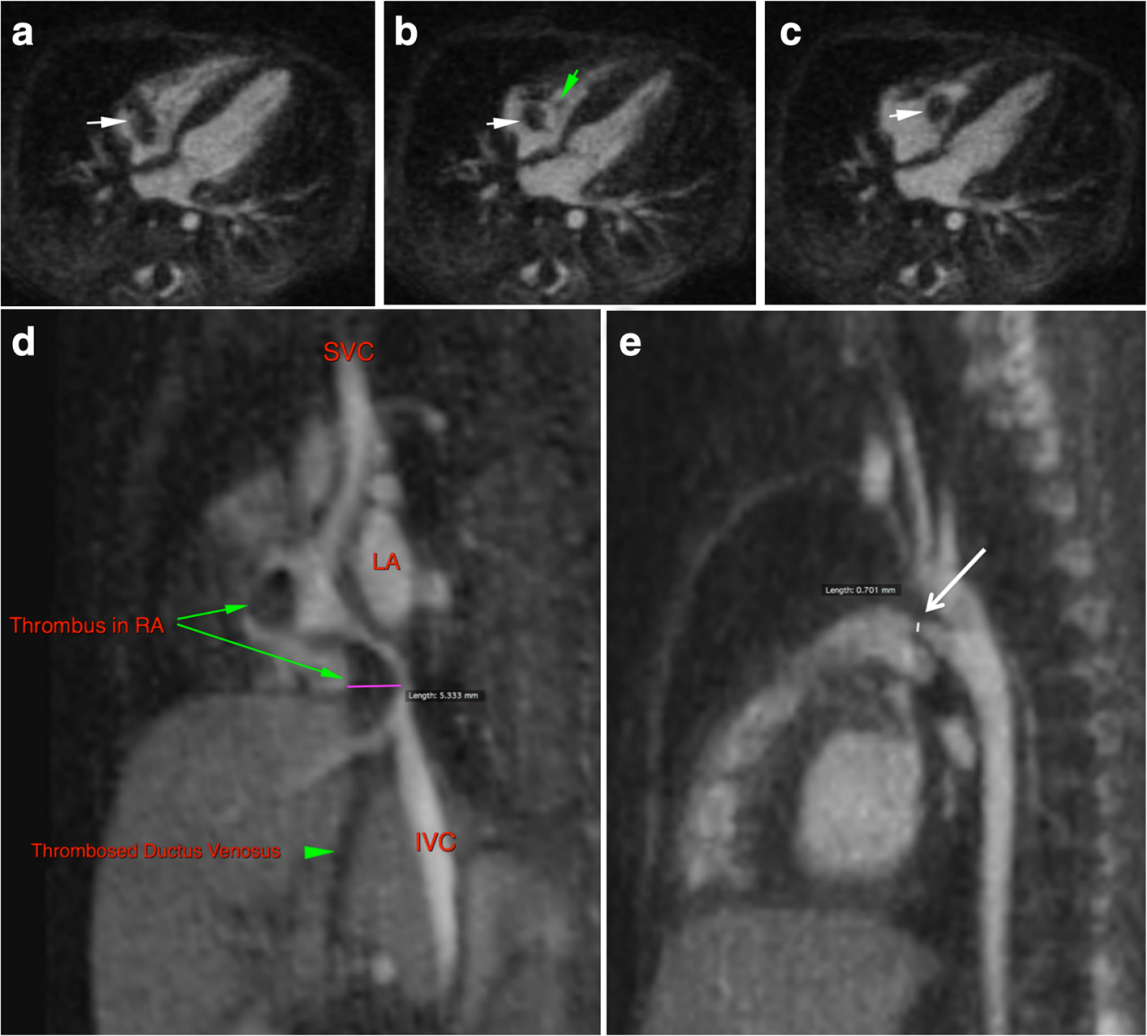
FE-MUSIC images of a twenty-one-day old boy (1.1 kg) with a patent ductus arteriosus (a-e). A mobile right atrial mass of uncertain etiology was noted on echocardiography after birth. Three of 8 frames from FE-MUSIC show a large mobile mass (*white arrow*) in the right atrium (RA) abutting the tricuspid annulus and valve leaflets (*green arrow*). The mass has to-and-fro motion and connects via a thin stalk to thrombus (d, Additional file 3: online video 1a) in the inferior vena cava, which in turn, is continuous with thrombus in the ductus venosus originating from an infected umbilical vein catheter. A patent ductus arteriosus measuring 700 microns at its waist is shown bridging the pulmonary artery and descending aorta in the FE-MUSIC image (e, Additional file 3: online video 1b)
